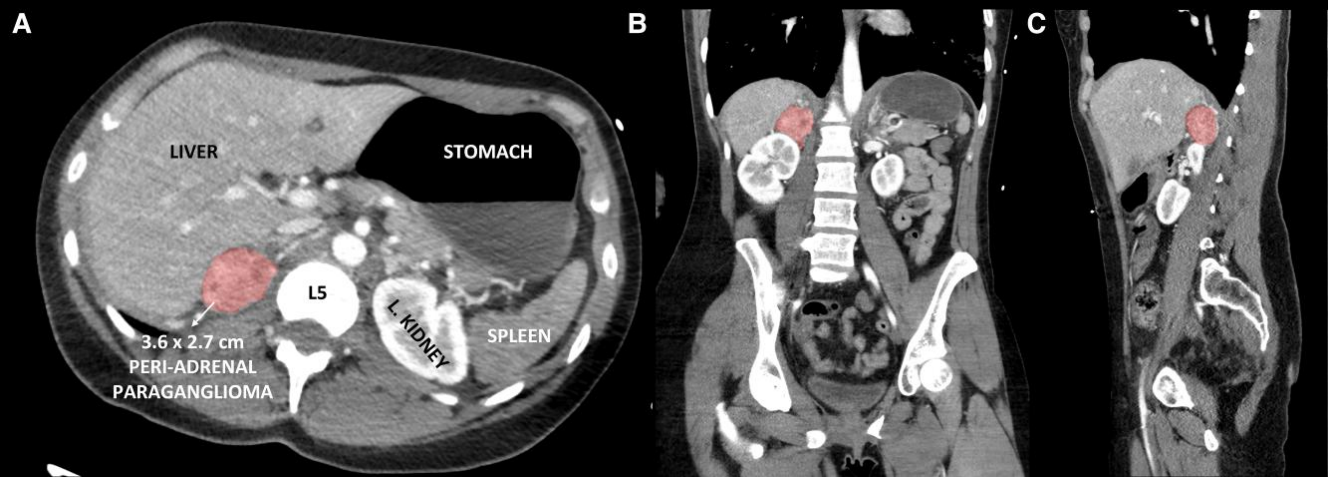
Computed tomography image of the 3.6 × 2.7 cm right-sided adrenal mass in coronal (*A*), axial (*B*), and sagittal (*C*) cuts.
